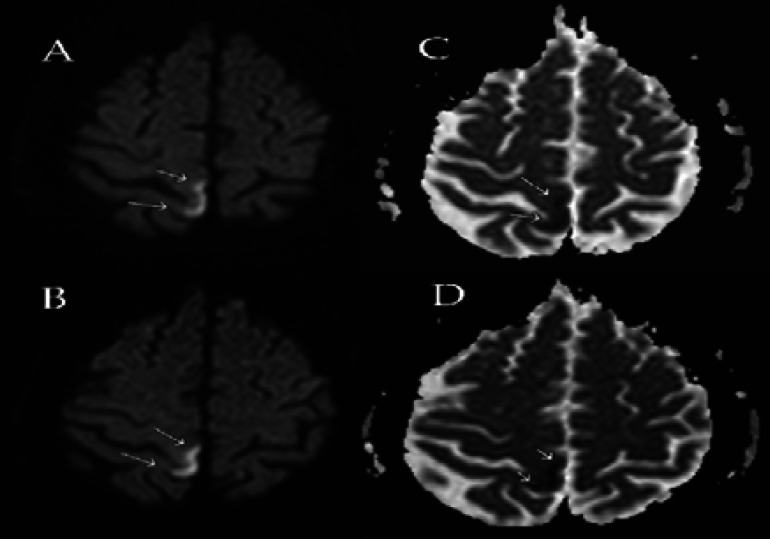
Brain magnetic resonance imaging (MRI) showing a right cortical parietal lesion hyperintense in diffusion weighted imaging sequence (A and B) and hypointense in apparent diffusion coefficient (ADC) mapping (C and D) compatible with acute ischemic stroke
